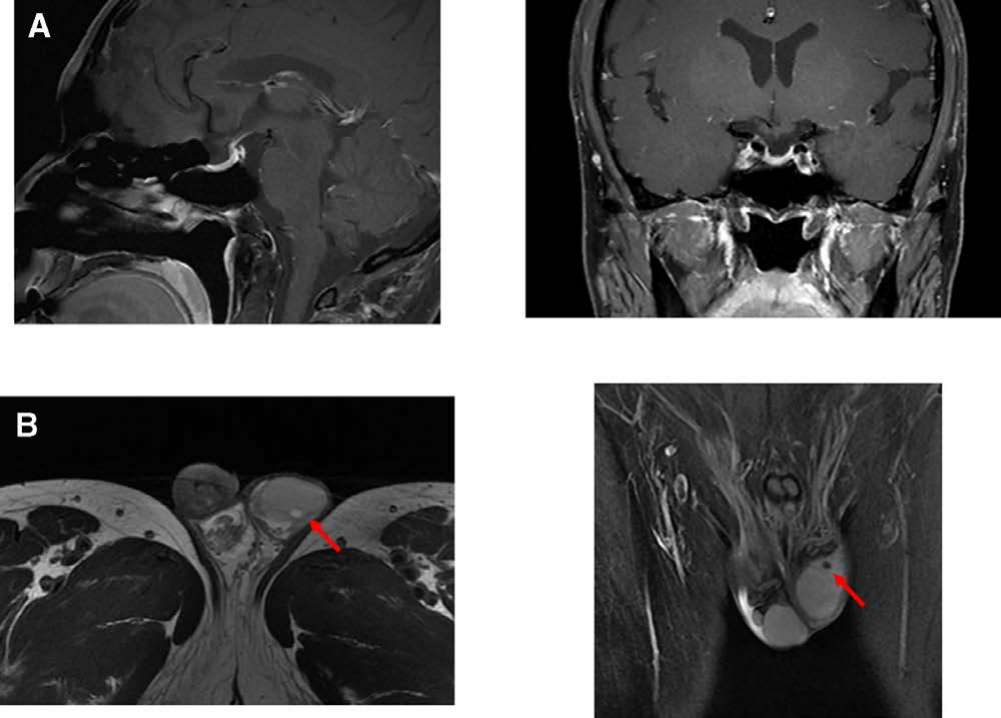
Imaging findings of the pituitary gland and left testis. (A) Contrast-enhanced pituitary MRI demonstrating an empty sella turcica. (B) Contrast-enhanced testicular MRI identifying a space-occupying lesion in the left testis (red arrow). MRI = magnetic resonance imaging.
